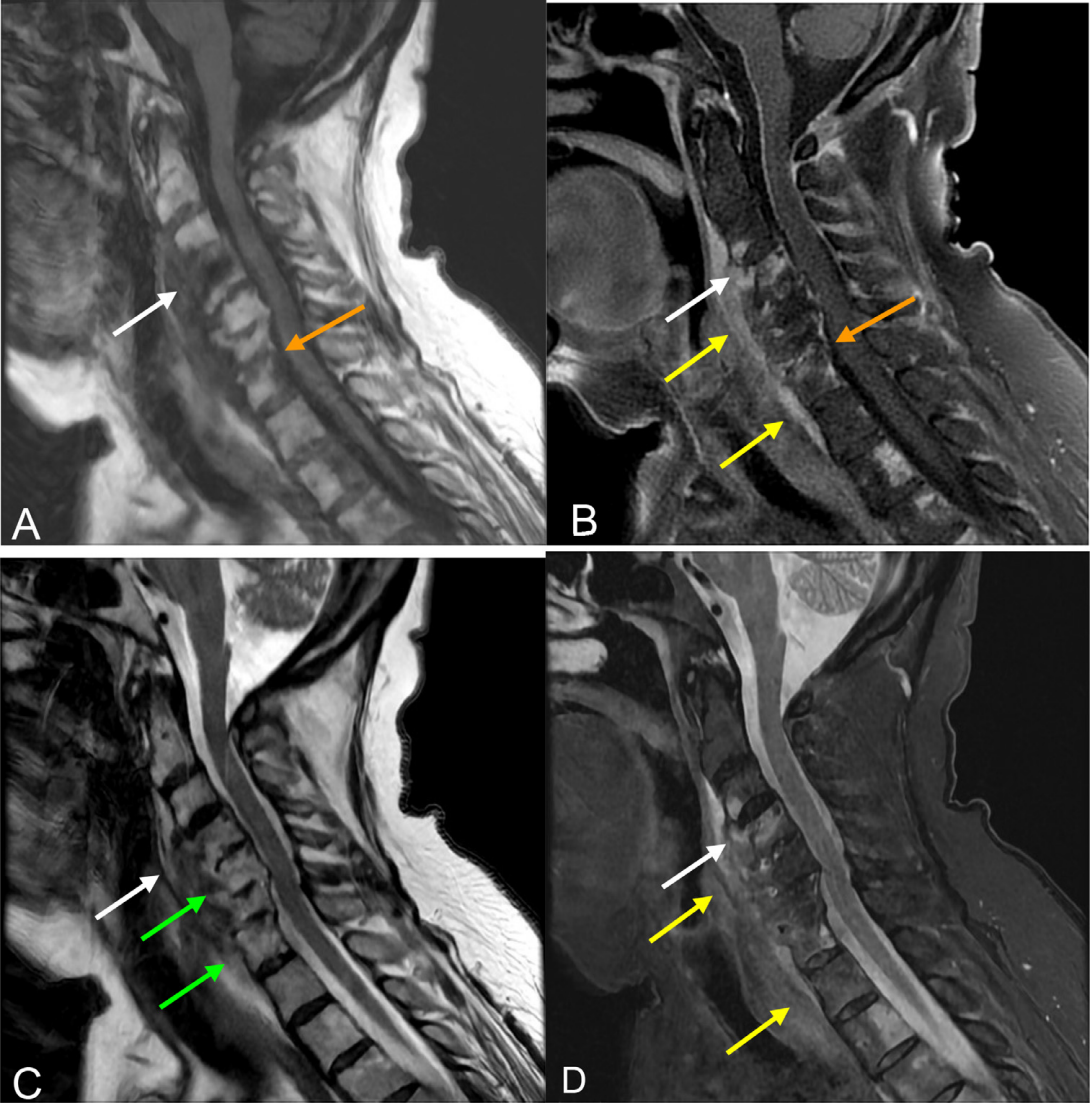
MRI cervical spine sagittal: (A) T1, (B) T1 fat-saturated (FS) post contrast, (C) T2, (D) STIR. (A-D) Additional skipped enhancing and destructive vertebral abnormalities were noted within the cervical spine primarily involving the endplates of C3-C4 (white arrow) and C6-C7 (orange arrow) (B&D) with relative sparing of T2 hypointense discs at the affected levels (green arrows). (D) Associated paravertebral edema which extends inferiorly along the anterior longitudinal ligament to the upper thoracic spine (yellow arrows).
